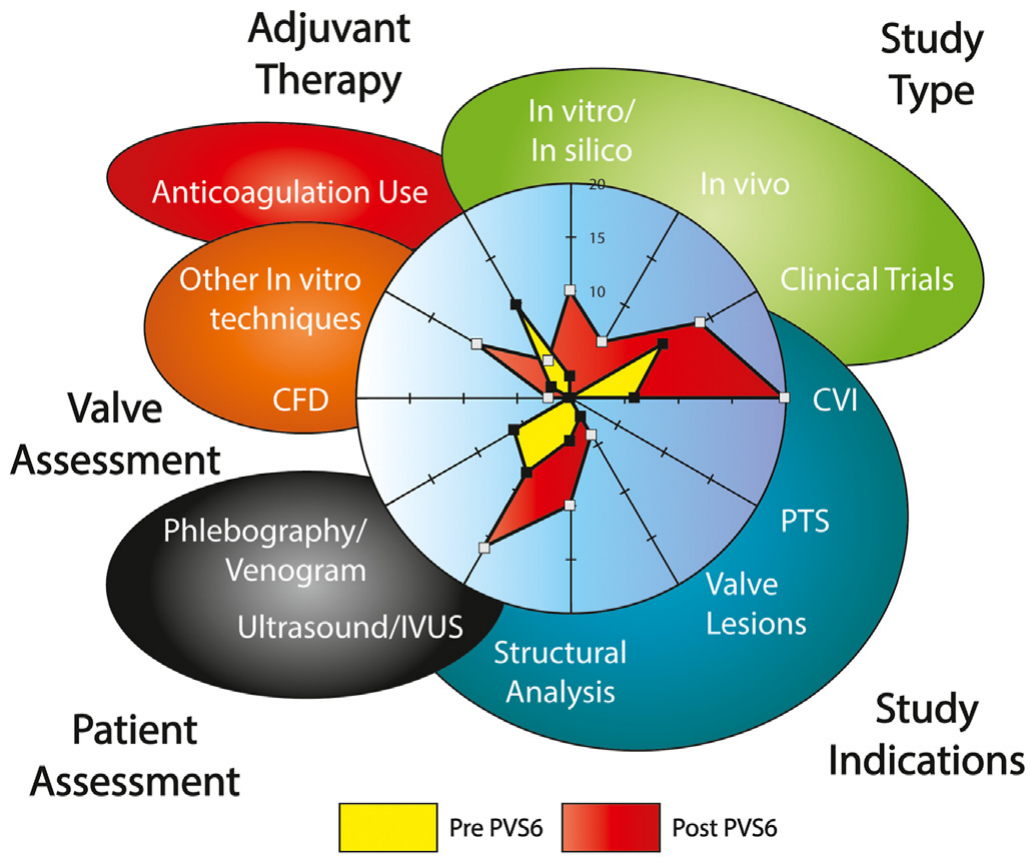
Artificial venous valve (AVV) study type, indication, preclinical and clinical valve assessment, and anticoagulation reported use. *CFD*, Computer fluid dymanics; *CVI*, chronic venous insufficiency; *IVUS*, intravascular ultrasound; *PTS*, post thrombotic syndrome.
